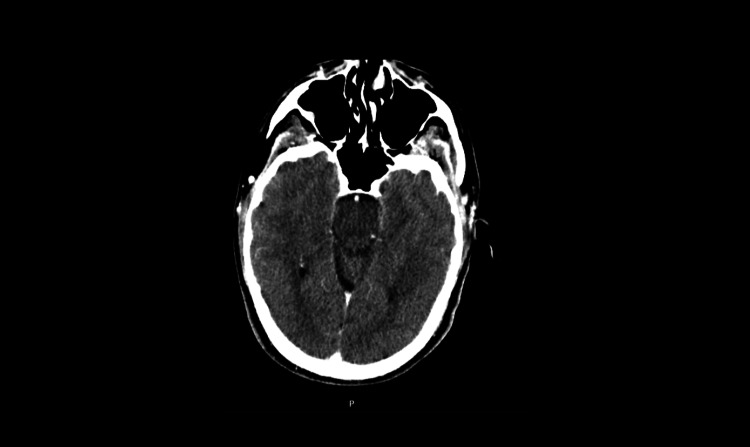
The initial CT head image was reported as unremarkable.
